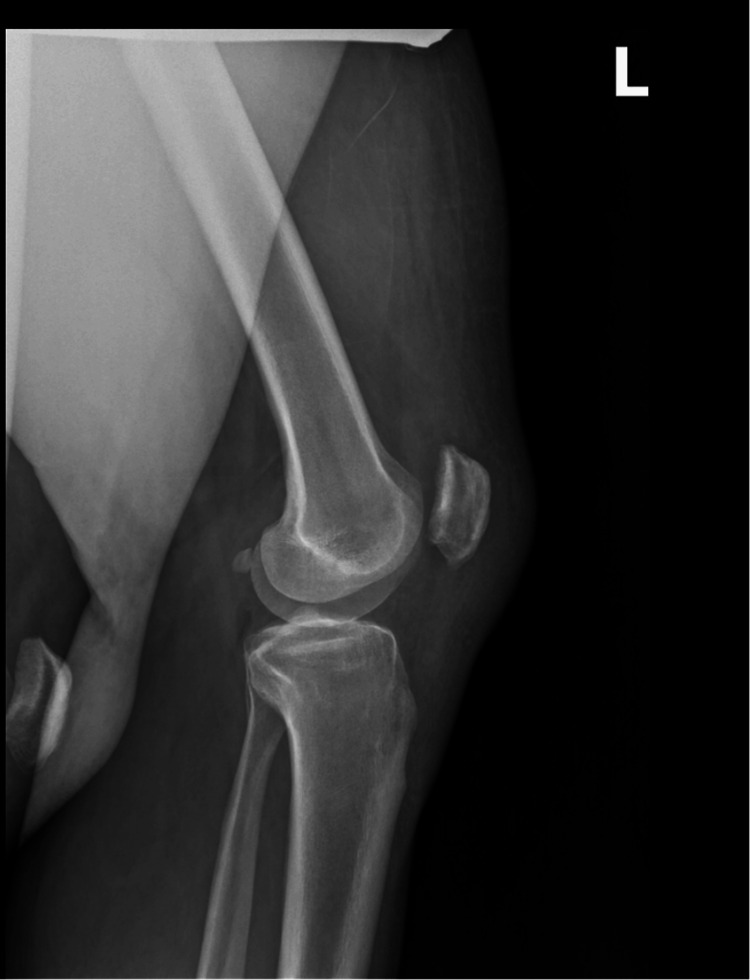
Postoperative lateral radiograph of the left knee Lateral radiograph obtained postoperatively demonstrates restored patellar height and alignment with no hardware or bony abnormalities noted. Findings are consistent with a successful extensor mechanism reconstruction.
